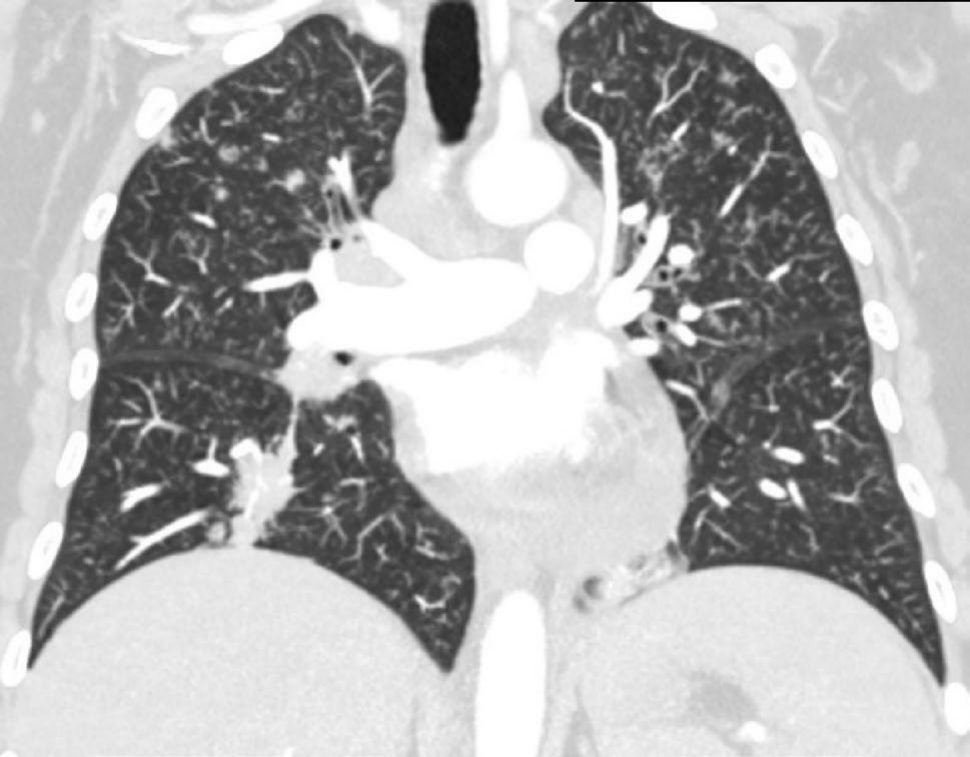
Coronal reconstruction of contrast-enhanced chest CT demonstrates multiple pseudonodular lesions in both lung fields, predominantly in the upper lobes, with consolidation in the right lower lobe
